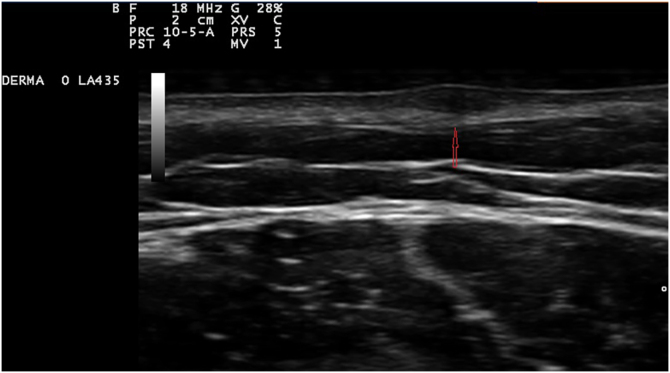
Ultrasonography shows a hypoechoic lesion located in deep dermis (arrow)
